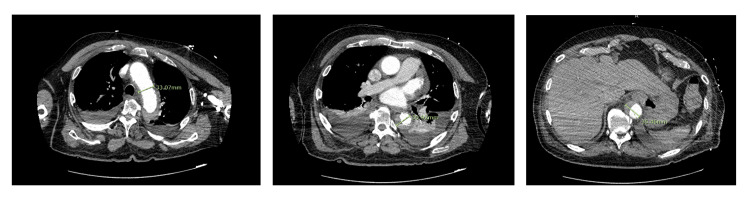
(Left to right) CT angiography with axial cut showcasing the patient’s aortic intramural hematoma (demarcated in green) extending essentially from the aortic root to just cranial to the renal arteries although without extent into any major branch vessels.
